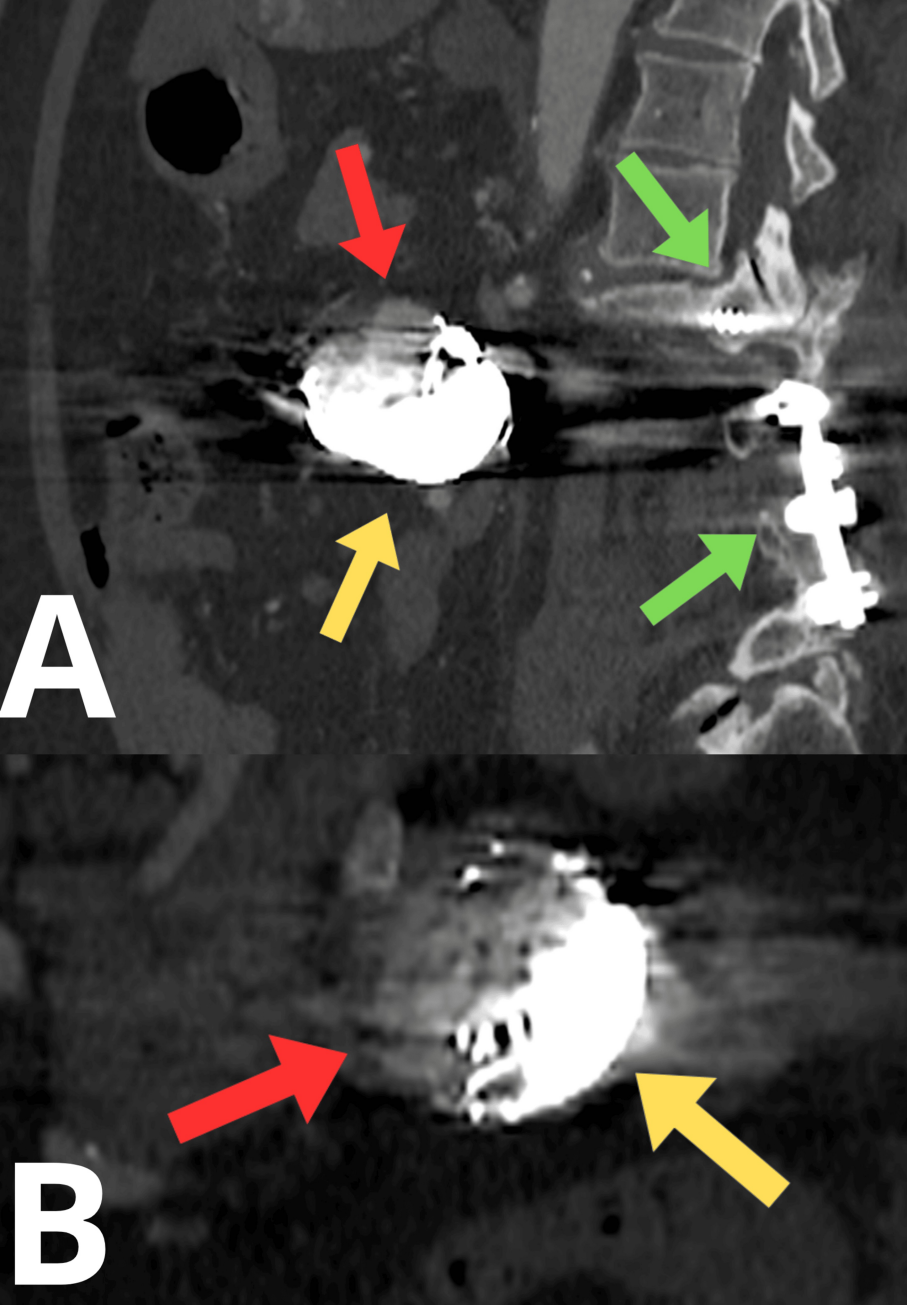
Angio-CT scan during follow-up. (A) Follow-up in April 2019. (B) Follow-up in February 2021. The yellow arrow indicates embolization coils. The red arrow indicates a filling aneurysmal sac. The green arrow indicates spinal screws and lumbar stabilization.
